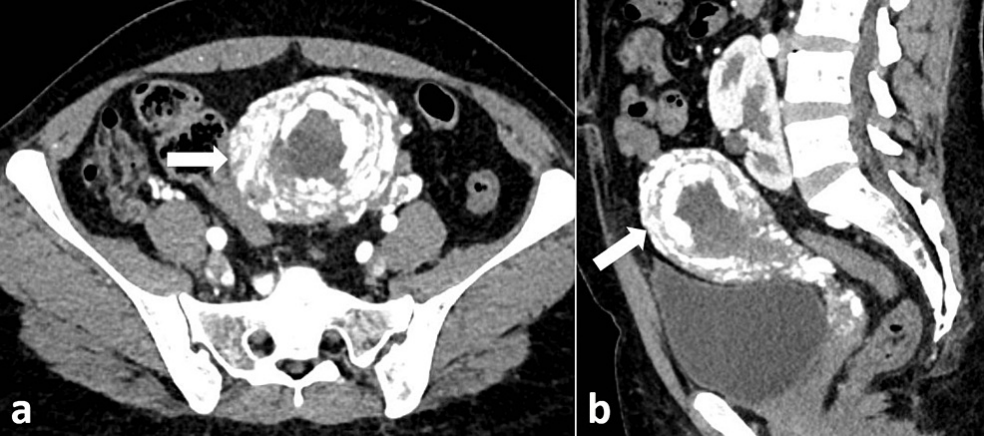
CT images of the patient with AVM CECT axial (a) and sagittal (b) arterial phase images show AVM (white arrows) involving the anterior and posterior myometrium with hypertrophied bilateral uterine arteries. The left kidney is ectopic in location. AVM, arteriovenous malformation; CECT, contrast-enhanced CT
